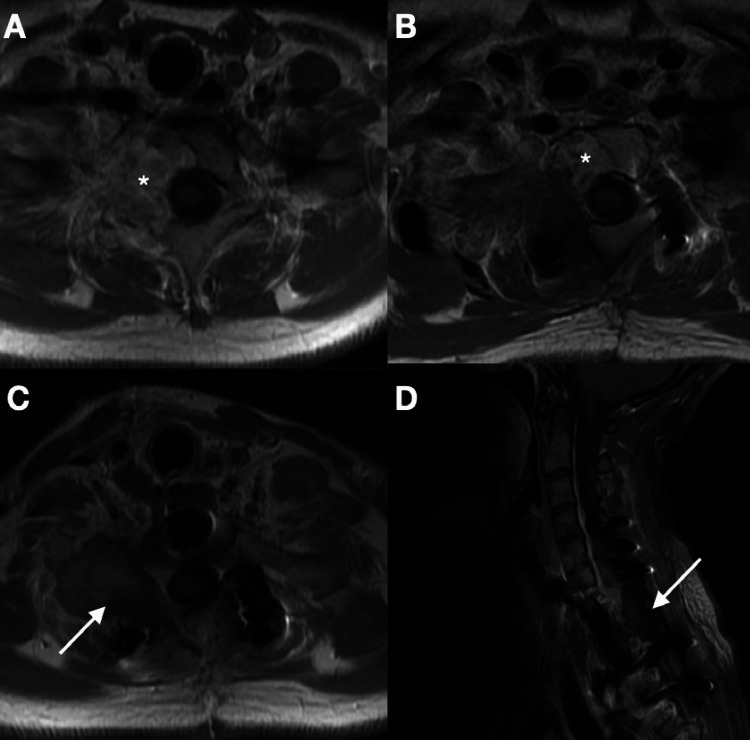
Postoperative images of the cervicothoracic junction tumor. (A) Axial preoperative magnetic resonance imaging (MRI). (B) After the first operation with the posterior approach, the axial MRI image showed wide resection of the tumor in the posterior area. Axial MRI (C) and sagittal MRI (D) showing complete excision of the tumor after anterior surgery, the second surgery. *: the tumor. White arrow: tumor lodge.
